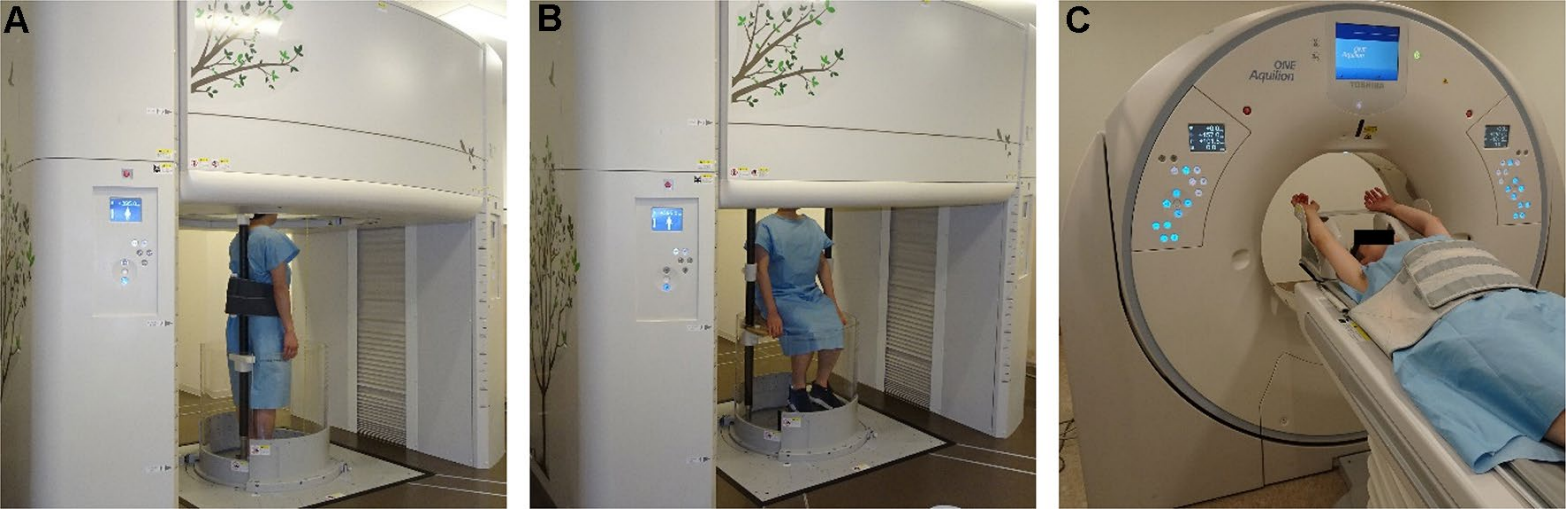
Upright CT examination in the standing position (A), upright CT examination in the sitting position (B), and conventional CT examination in the supine position (C). Upright CT examinations in the standing position (A) and sitting position (B) were performed with the subject’s arms down during both deep inspiration breath-hold and expiration breath-hold. Conventional CT in the supine position (C) was performed with the subject’s arms raised during both deep inspiration breath-hold and expiration breath-hold. *CT* computed tomography.
